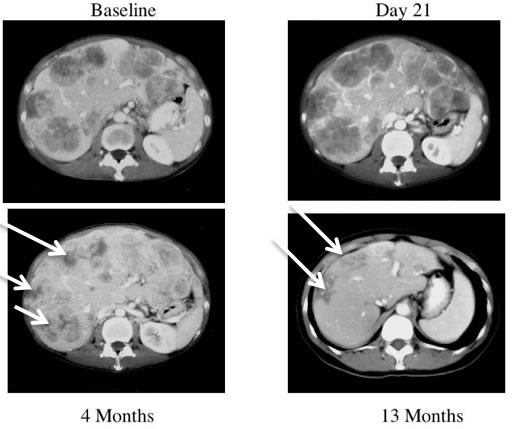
CT scans of a patient receiving ONYX-015 This patient received treatment with ONYX-015 on days 1 and 8 and CT scans are shown from baseline, Day 21, 4 months and 13 months. The baseline CT scan of this patient demonstrates extensive disease in all lobes of the liver. The CT on day 21 demonstrates therapy-related enlargement of the tumor masses so-called pseudoprogression. The patient continued with monthly treatment with ONYX-015 in combination with 5-FU/leucovorin and improvement of the tumor masses (white arrows) was observed at 4 months. The masses (white arrows) have largely resolved by 13 months [88].
